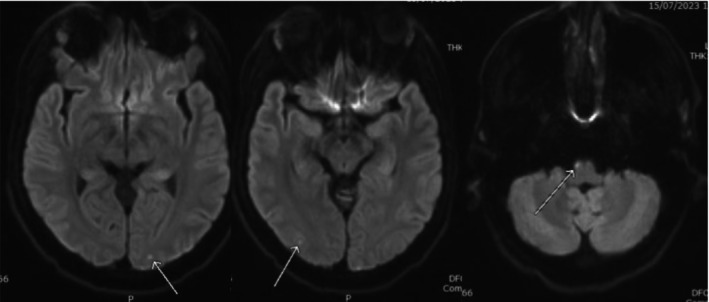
DWI images showing multiple tiny foci of restricted diffusion in the right medullary pyramid as well as bilateral occipital cortex suggestive of acute infarcts.
